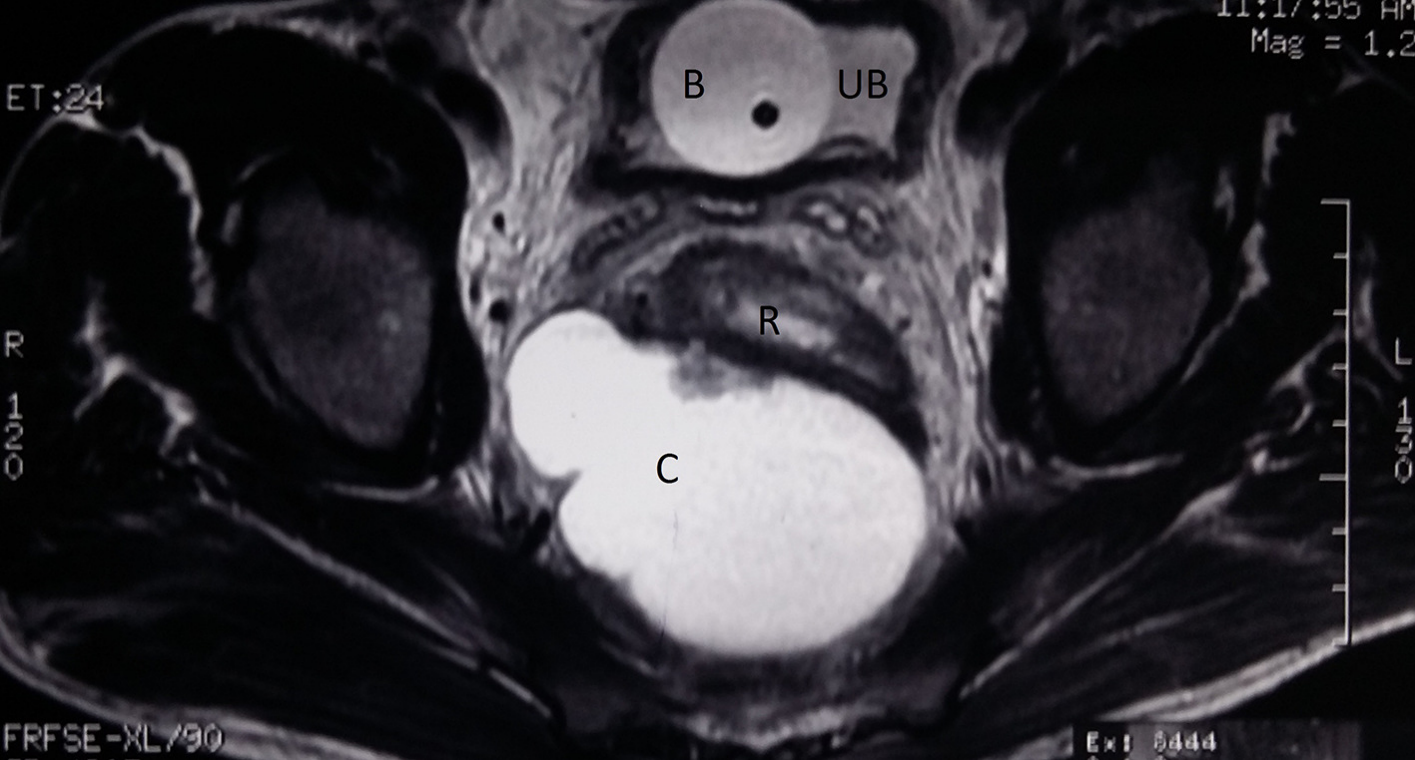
MRI axial: axial and sagittal *T*_2_ weighted images show the septa and a mural nodule within. The cyst wall appears to be tightly adherent to the posterior wall of the rectum (R). Urinary bladder (UB) with catheter bulb (B) in situ noted. S, sacrum; P, pubic bone.
